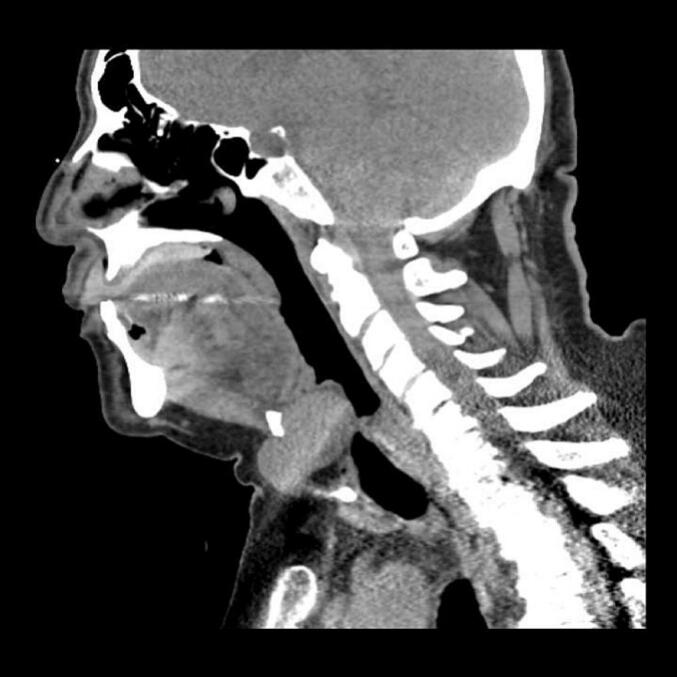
Sagittal, contrast-enhanced computed tomography (CT) image of Patient 2 shows a cystic lesion with upward growth posterior to the hyoid bone.
